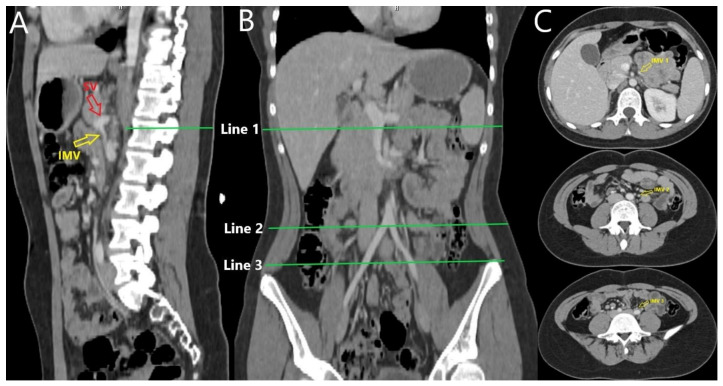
Measurement levels of the inferior mesenteric vein (IMV) in a 14-year-old girl. (A) Sagittal computed tomography (CT) image demonstrating the termination of the IMV into the splenic vein (SV). (B) Coronal reconstruction showing the three predefined measurement levels: IMV1 (terminal segment, line 1), IMV2 (aortic bifurcation level, line 2), and IMV3 (pelvic brim level, line 3). (C) Corresponding axial CT images demonstrating the measurement of IMV caliber at each level. IMV diameters were measured perpendicular to the long axis of the vein from outer wall to outer wall.
